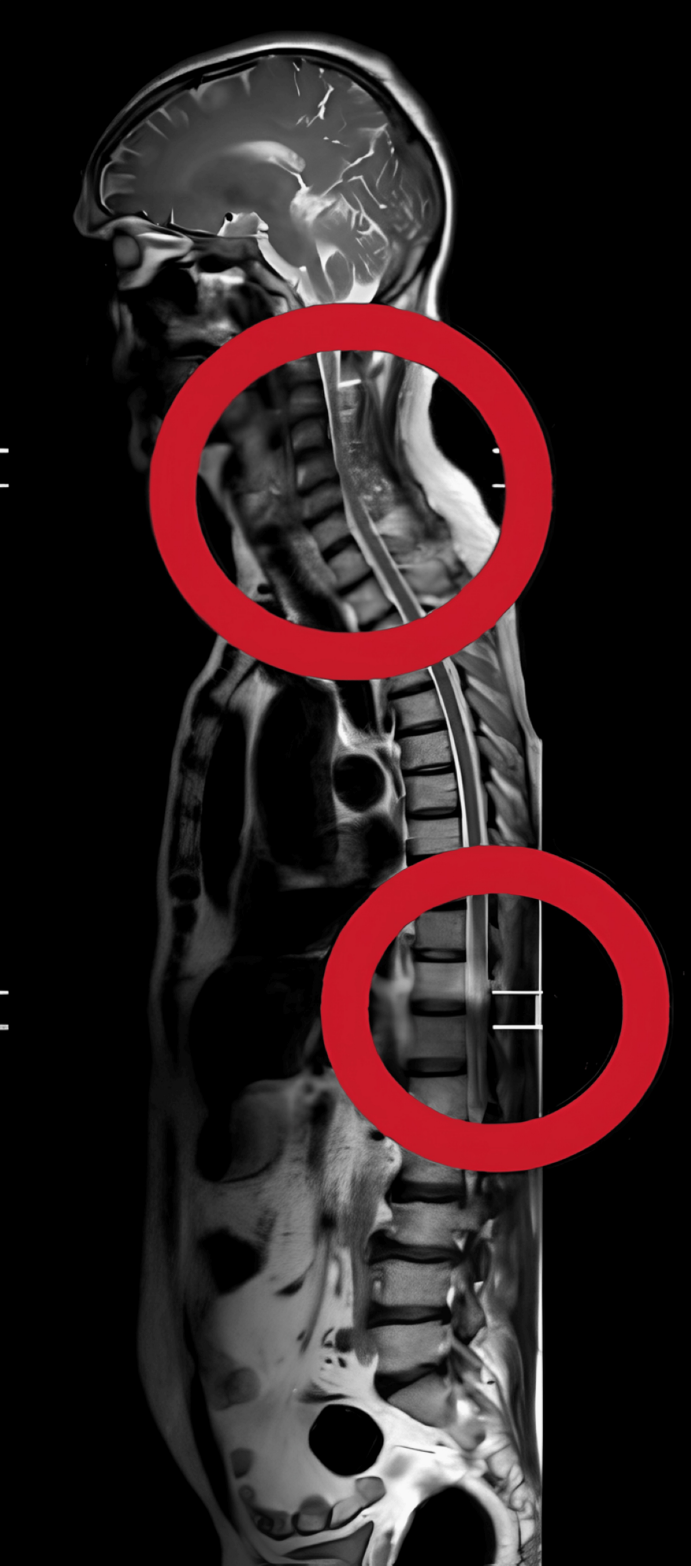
MRI showing compression at the cervical and lumbar region (shown with red circles)
